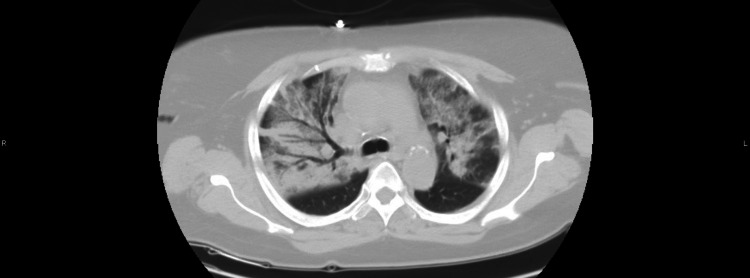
CT Chest w/o contrast image 1
